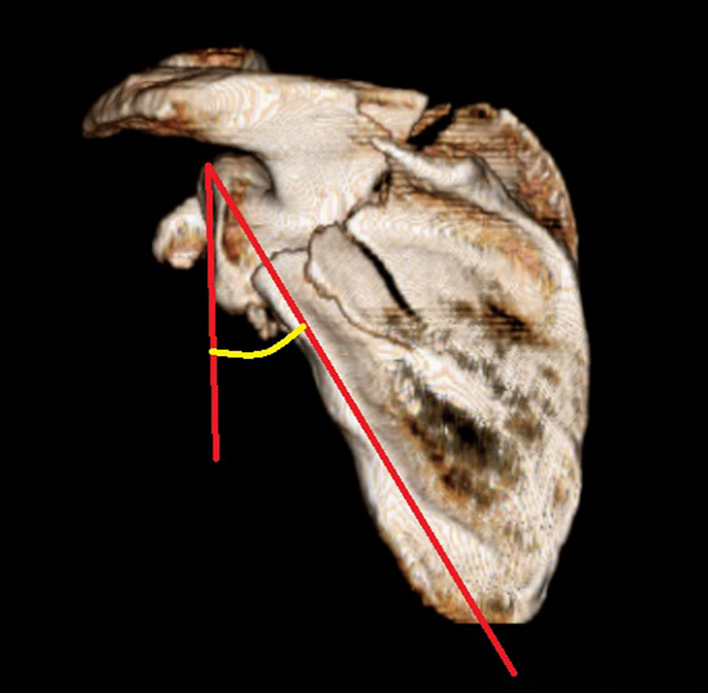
The glenopolar angle as measured on 3D CT reconstruction. The apex created by *two lines* extending from the superior glenoid pole to the mid-point of the inferior angle and inferior glenoid pole determine the glenopolar angle
